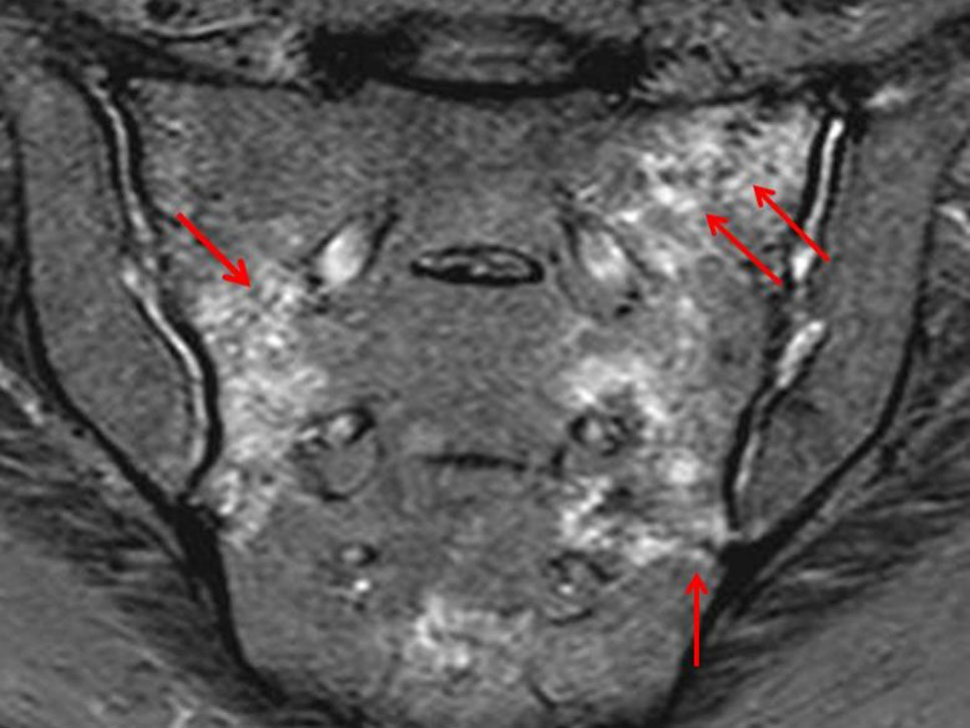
Image of a bilateral sacral fracture, on the right a Denis type 1 fracture zone and on the left with a Denis type 1–2 fracture zone, in the coronal MRI image with strong T2 weighting and partial fat suppression. The bilateral bone marrow oedema is clearly visualised; the* red arrows* mark the fracture lines (colour figure online)
